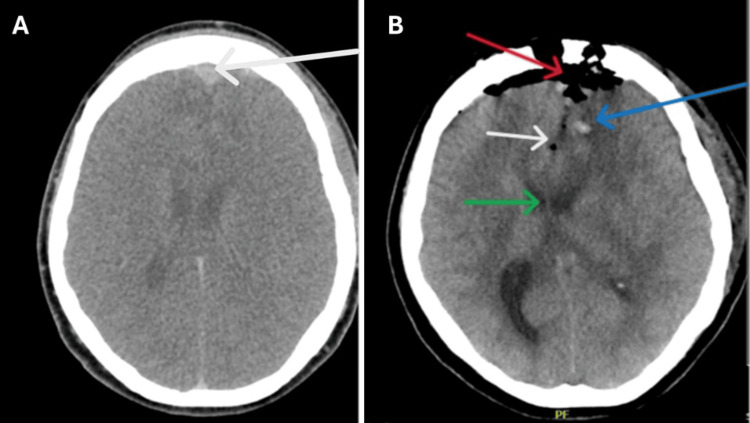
Postoperative CT brain. (A) CT brain shows evidence of a thin subdural density collection over the frontal lobe (white arrow). (B) CT brain image shows a frontal craniotomy with pneumocephalus (red arrow), small-volume haemorrhage (blue arrow), intracranial air (white arrow), and residual midline shift to the right (green arrow). CT, Computed Tomography
